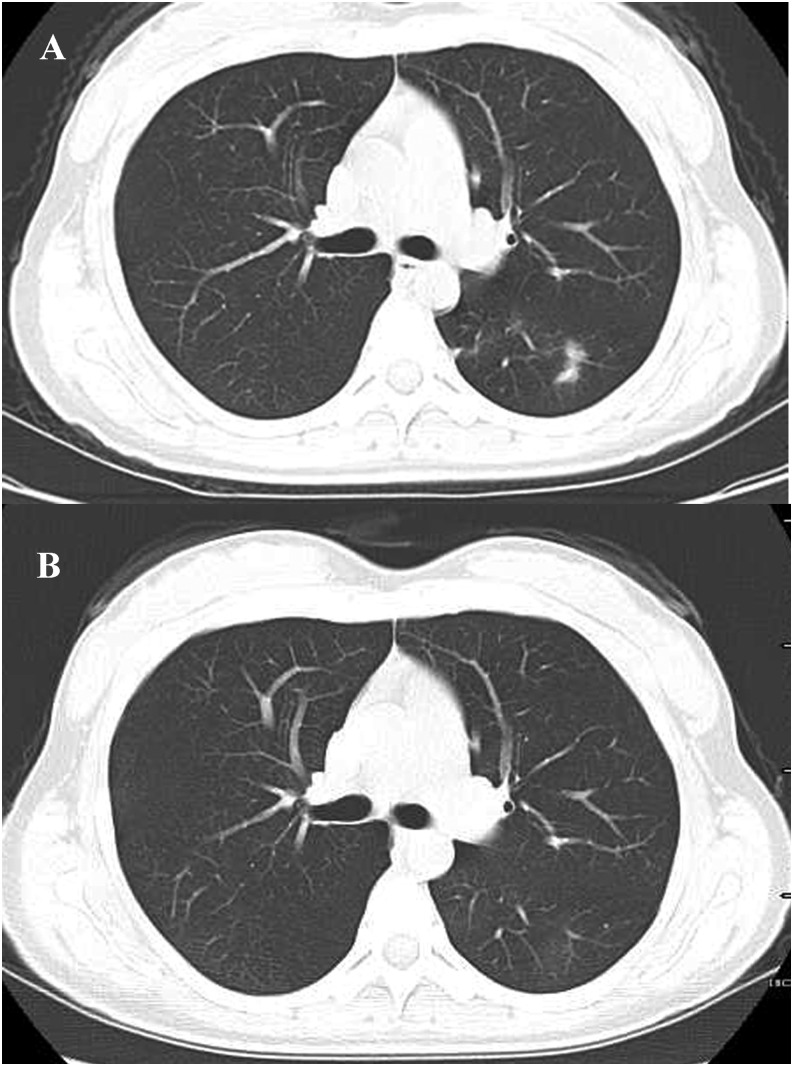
CT scan showed ill-defined reticulonodular infiltrates in both lungs (A), and disappeared 10 days after treatment (B).
